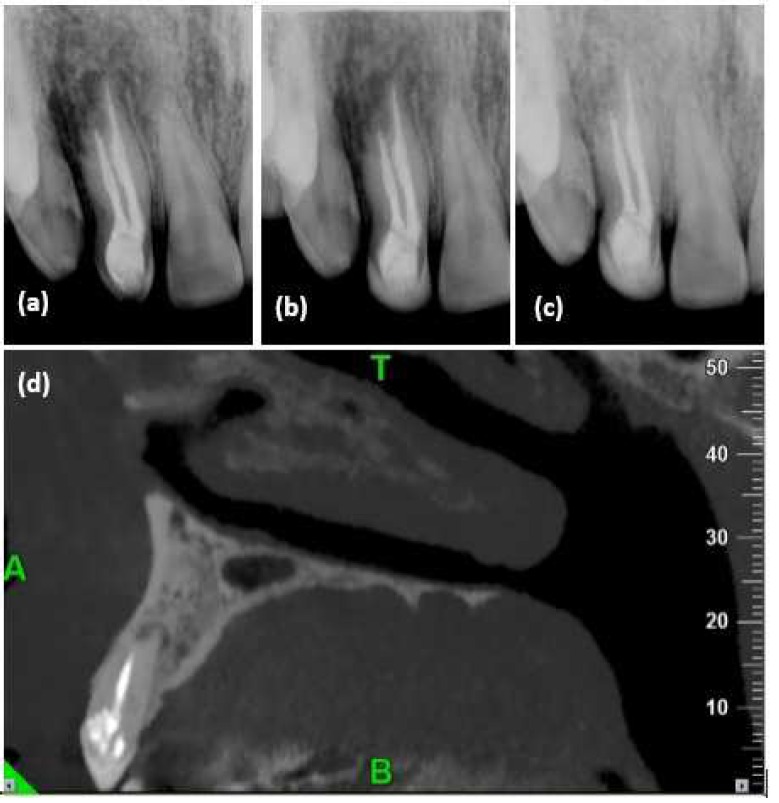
Postoperative radiographies; *A)* immediately after treatment; *B*) one-year and *C and D*) two-year follow ups
